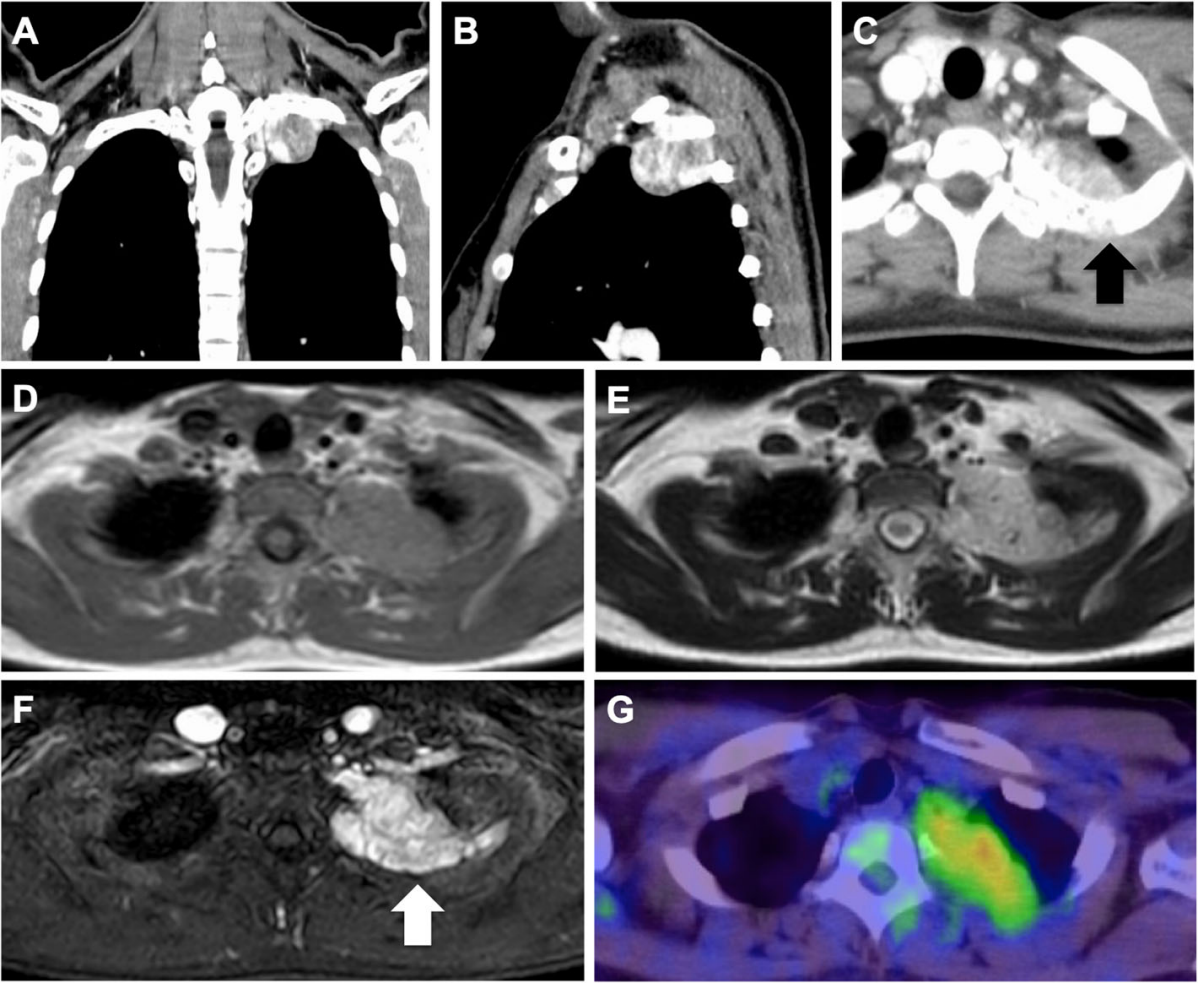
a Contrast-enhanced computed tomography revealing a solitary nonhomogeneous 4-cm mass in the posterior mediastinum. b, c The mass invaded the left 2nd rib (black arrow). d, e Magnetic resonance imaging (MRI) showing that the signal inside the mass was representative of muscle tissues equivalent to that on the T1-weighted image and hyperintense on the T2-weighted image. f Contrast-enhanced MRI showing similar signal changes and contrast effects on the dorsal side of the second rib in contact with the tumor. Bone infiltration is noted (white arrow). g Positron emission tomography/computed tomography scan with F-18 fluorodeoxyglucose showing standardized uptake values of 2.69
